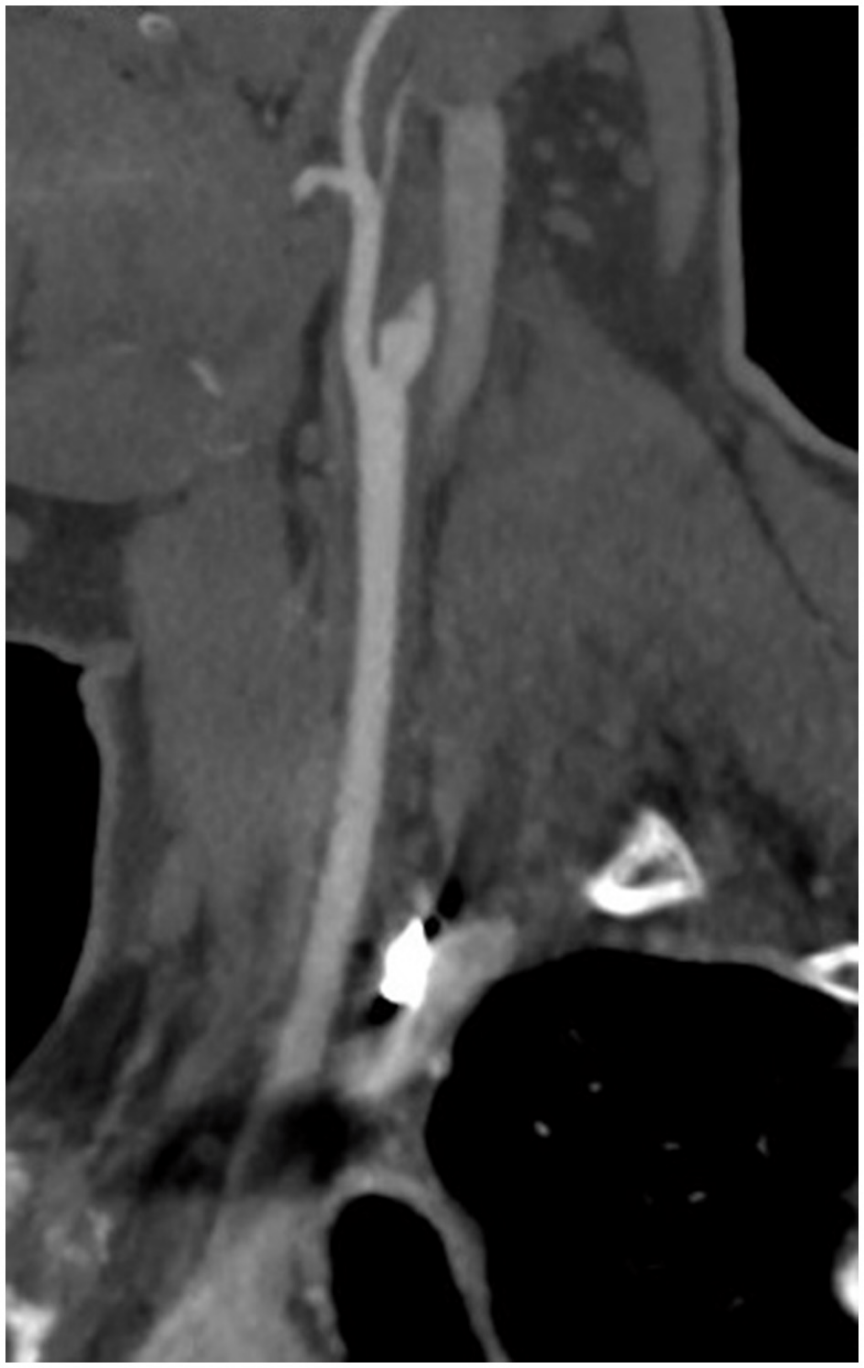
CT angiography demonstrating the “flame sign,” usually indicative of arterial dissection, in the right internal carotid artery.
